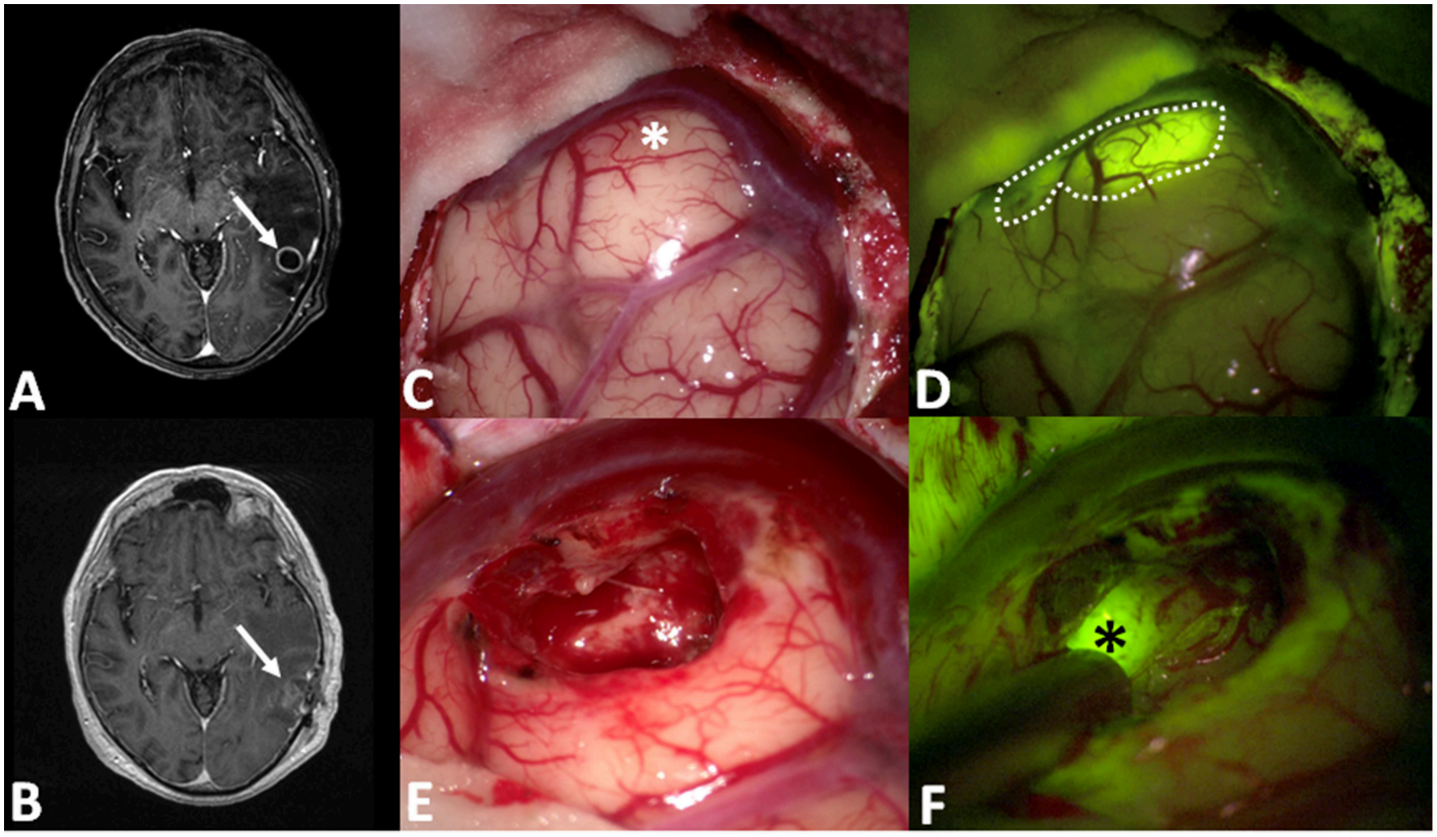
(A,B) Pre- and post-operative post-contrast axial T1-weighted scans showing a left temporal metastasis from lung cancer, completely removed. After dural opening (C), the Y560 filter (D) can highlight the superficial part of the metastasis, helping the surgeon in discriminating it from peritumoral brain parenchyma (dotted line in D), whereas under white light it is possible to appreciate a grayish, vanishing area (asterisk in C). During surgical resection (E,F), fluorescein helps the surgeon to identify residual pathological tissue (black asterisks in F), not clearly visible under white light (E).
